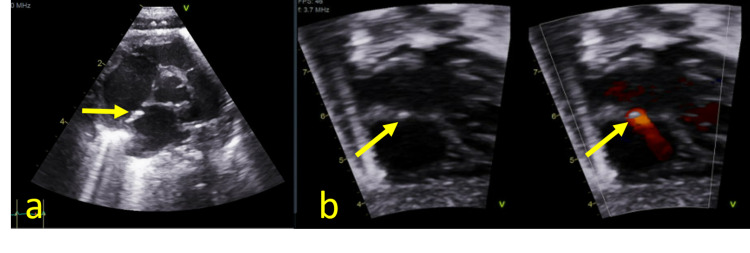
Transthoracic echocardiogram on day 32 with 5-chamber (a) and 4-chamber (b) views with grayscale and color Doppler demonstrating a hyperechoic focus along the interatrial septum (arrows)
